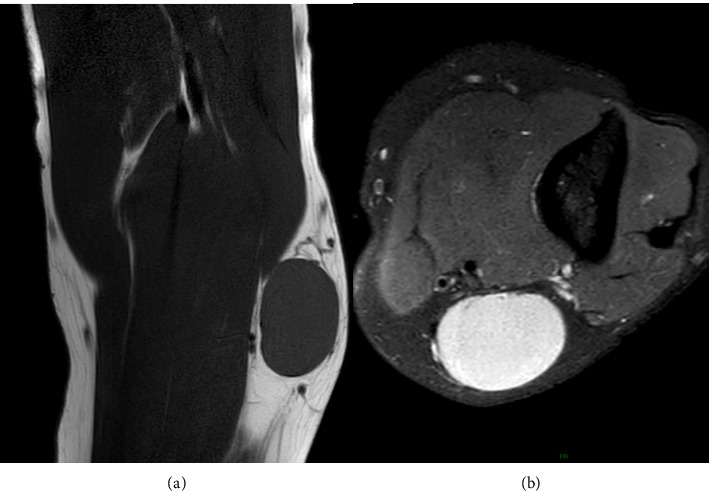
MRI exam. Coronal T1 TSE MRI sequence (a) demonstrating a hypointense, occupying space mass, localized in the upper para-epitroclear seat, that in the axial T2 TSE MRI sequence (b) appear homogeneously hyperintense.
